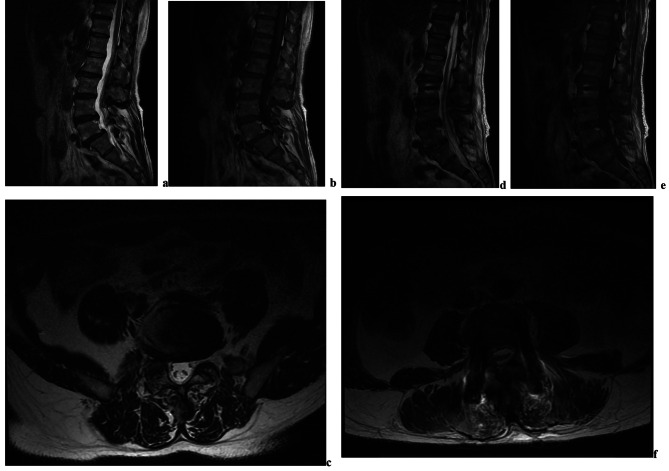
The ventrodorsal combined-type SSH. (a) preoperative T2-weighted sagittal magnetic resonance (MR) image; (b) preoperative T1-weighted sagittal MR image; (c) preoperative T2-weighted axial MR image; (d) postoperative T2-weighted sagittal MR image; (e) postoperative T1-weighted sagittal MR image; (f) postoperative T2-weighted axial MR image
